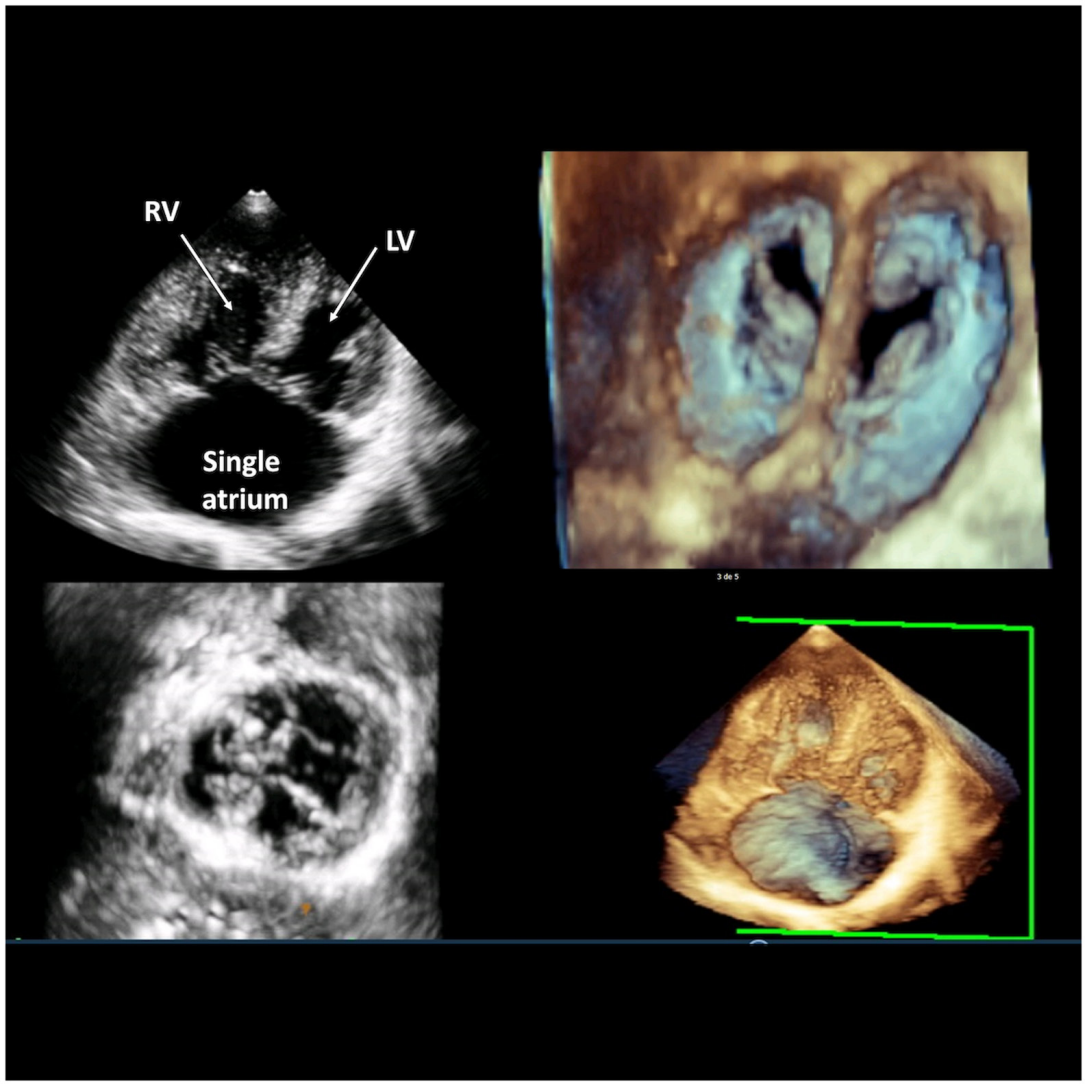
Two‐dimensional echocardiography images revealing common atrium/apical 4‐chamber view showing complete absence of interatrial septum. CA, common atrium; LV, left ventricle; RV, right ventricle.
